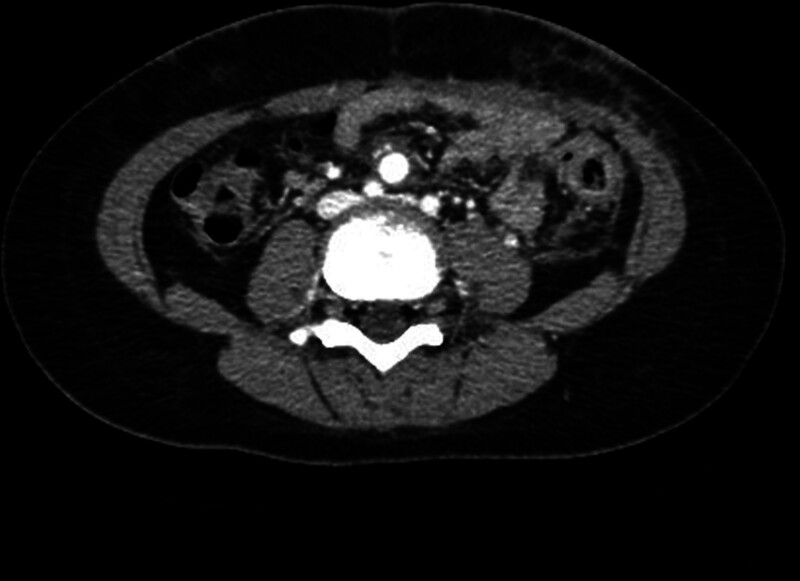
Follow-up abdominal CT scan after 2 months showing a highly enhancing round lesion (1 cm) in the mid-abdomen, suggesting a pseudoaneurysm of the ileal branch of the superior mesenteric artery.
